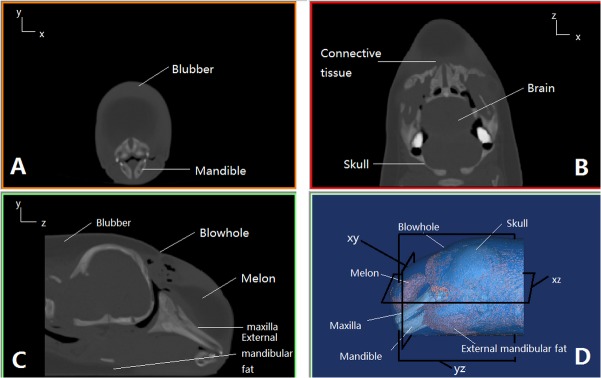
The 2-D and 3-D views of the finless porpoise’s head. (A) The axial cross section (xy, front view). (B) coronal cross section (xz, top view). (C) sagittal cross section (yz, lateral view). (D) 3-D reconstructed graph of the finless porpoise’s head by CT scan.
